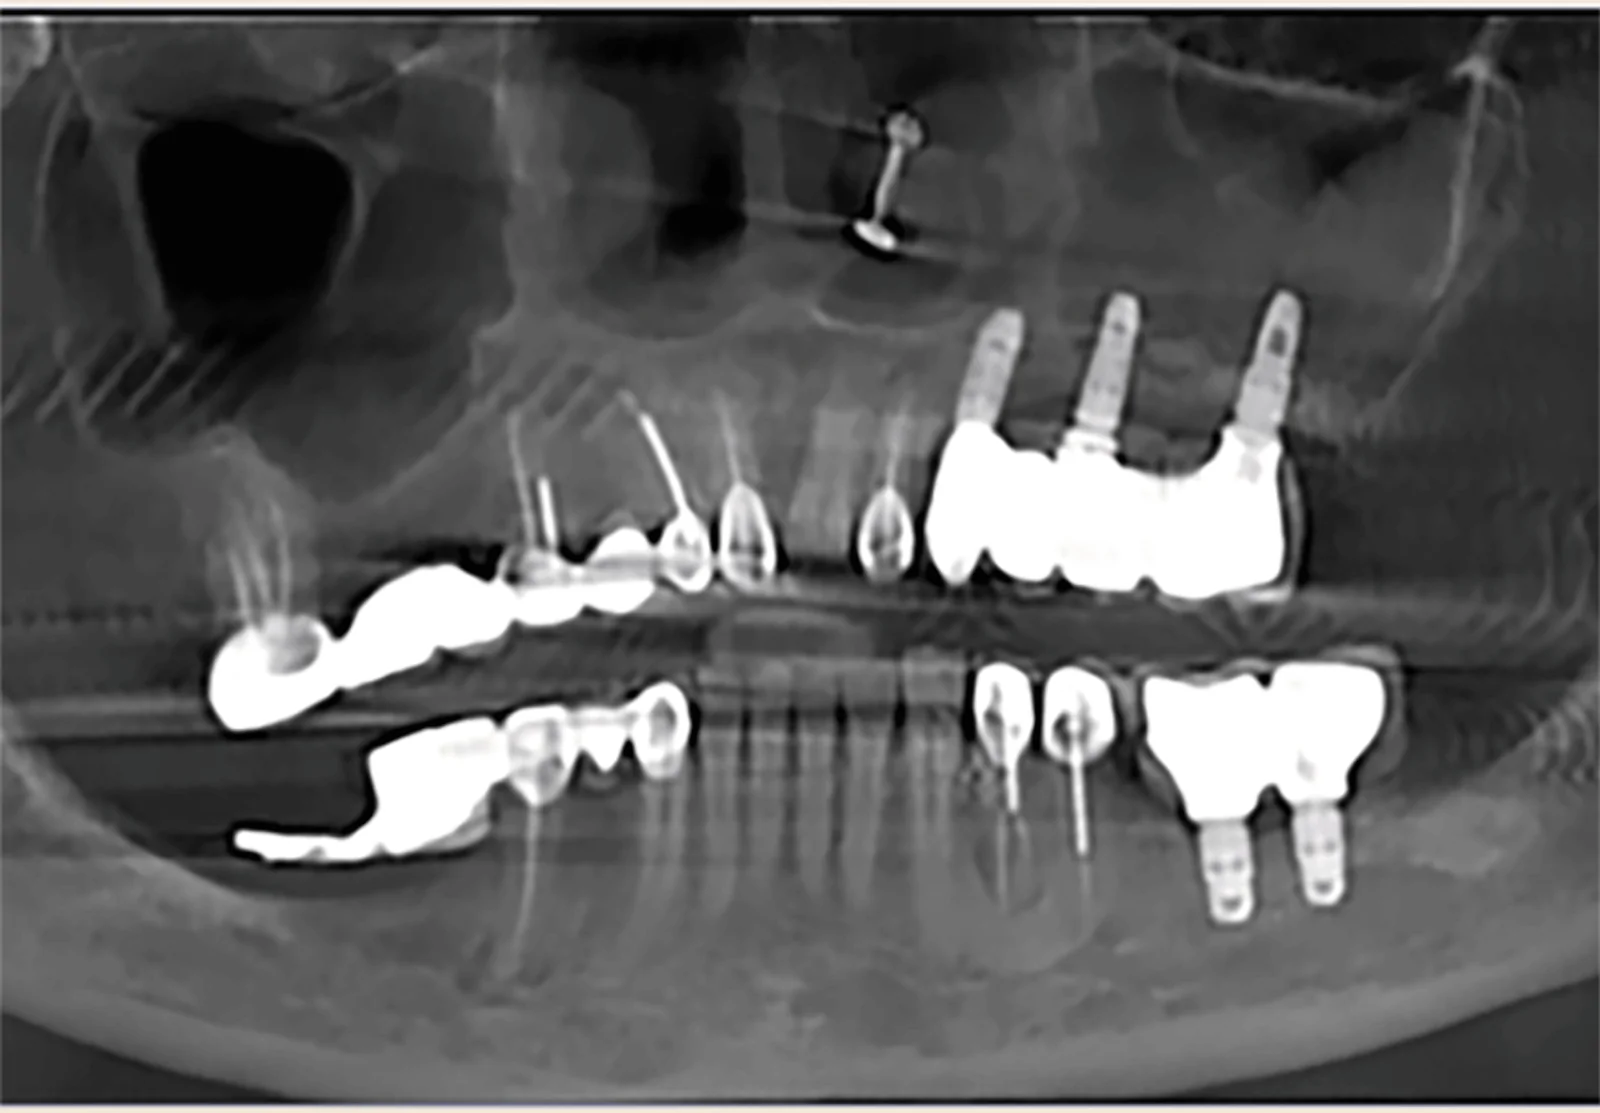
OPG X-ray taken one month after prosthesis delivery. OPG: orthopantomogram
